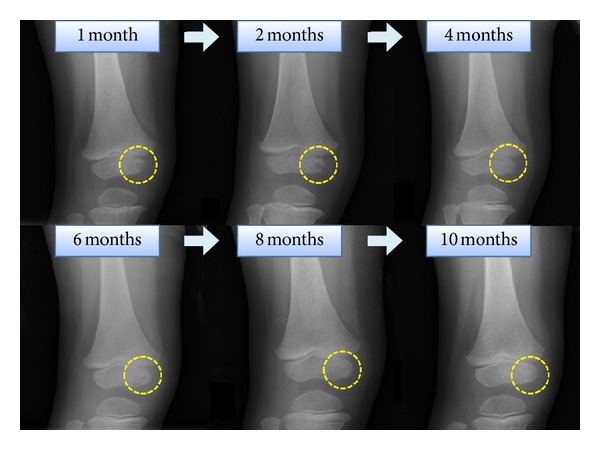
Radiograph at 1 month after onset of disease shows the radiolucency in the medial epiphyseal nucleus. Radiograph at 4 months shows the start of remodeling the epiphysis. Radiograph at 10 months returns almost normal findings.
